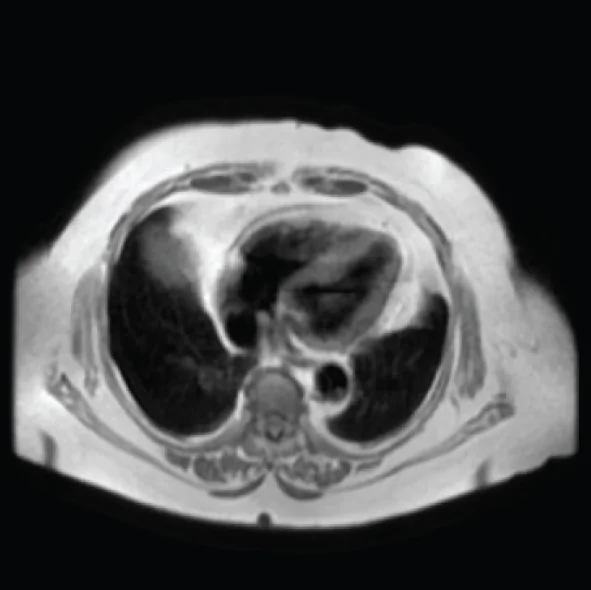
Cardiac MRI demonstrating prominent epicardial fat consistent with epicardial lipomatosis.
